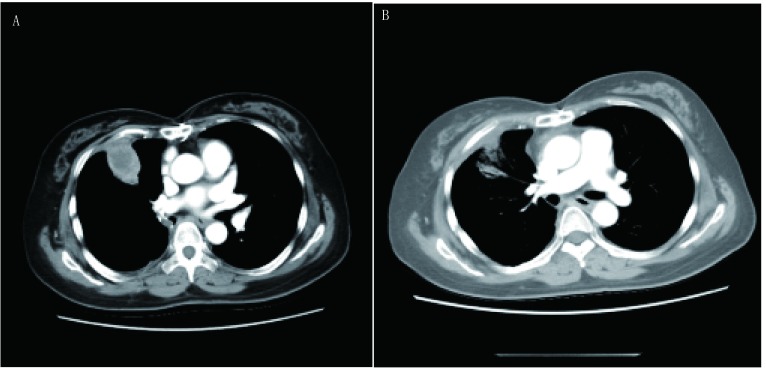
右肺平滑肌肉瘤累及左心房术后CT复查。A：术后15个月，肿瘤复发；B：化疗2个月后（术后17个月），肿瘤明显缩小。 Postoperative CT scan of the right lung leiomyosarcoma involving the left atrium. A:Tumor recurrence 15 months after operation; B:After 2 months of chemotherapy (17 months after the operation), the tumor was significantly reduced.
